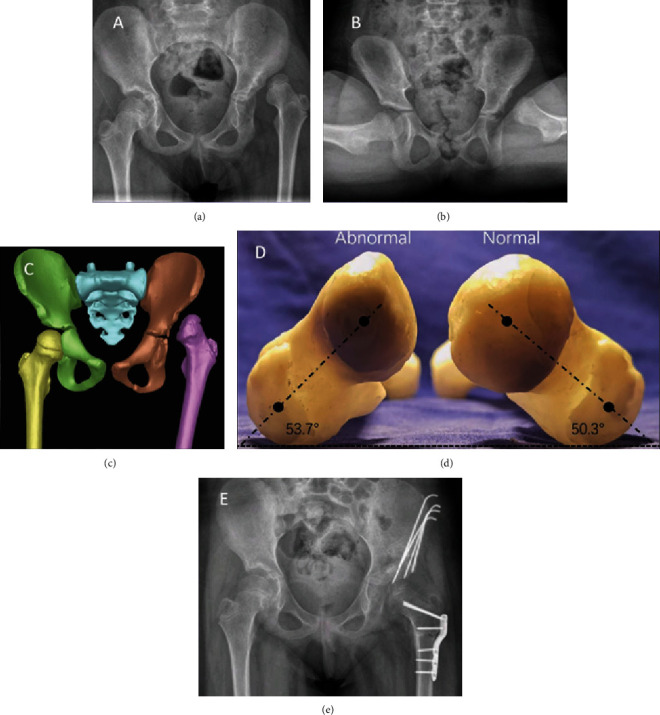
The patient was a 12-year-old female with left DDH and progressive left hip pain for 1 year. She underwent left pelvic osteotomy combined with proximal femoral shortening and varus rotating osteotomy. (a, b) Preoperative radiography of the pelvis showed dislocation of the left hip joint. (c) The pelvis 3D model showed dislocation of the left hip joint. (d) Measuring the femoral neck anteversion by the femoral model. (e) Anteroposterior radiography of the pelvis after 3-month postoperation, indicating the reduction of the hip joint.
